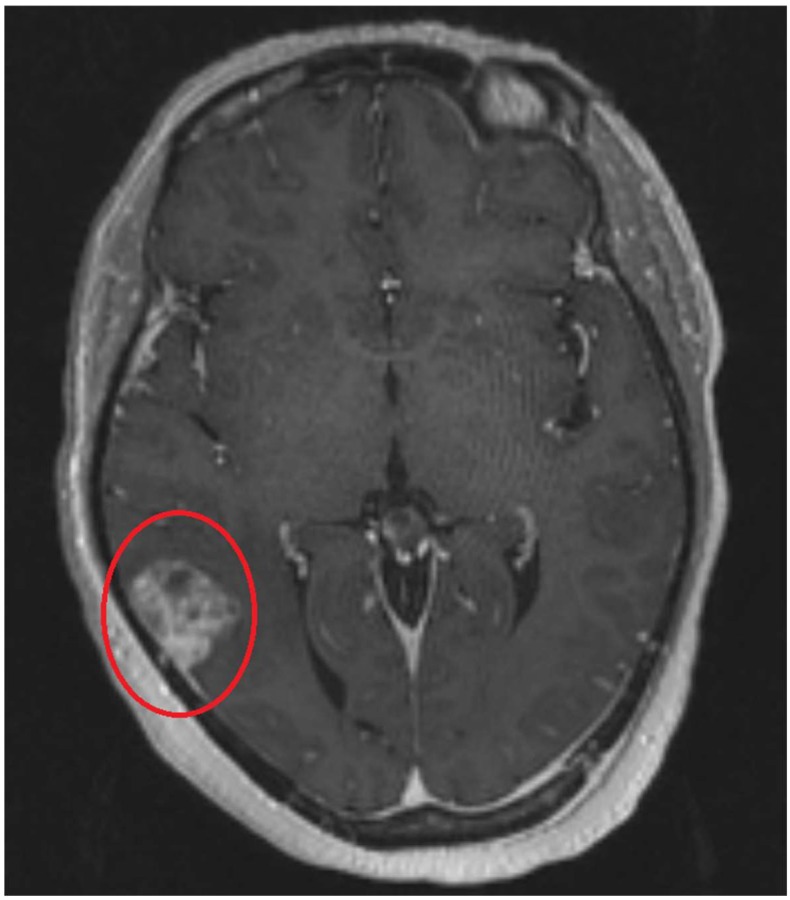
A T1 post-contrast axial magnetic resonance image of a contrast-enhancing tumor (circled in red). This patient is a 57-year-old female with metastatic breast carcinoma. The image and presentation are consistent with a brain metastasis.
